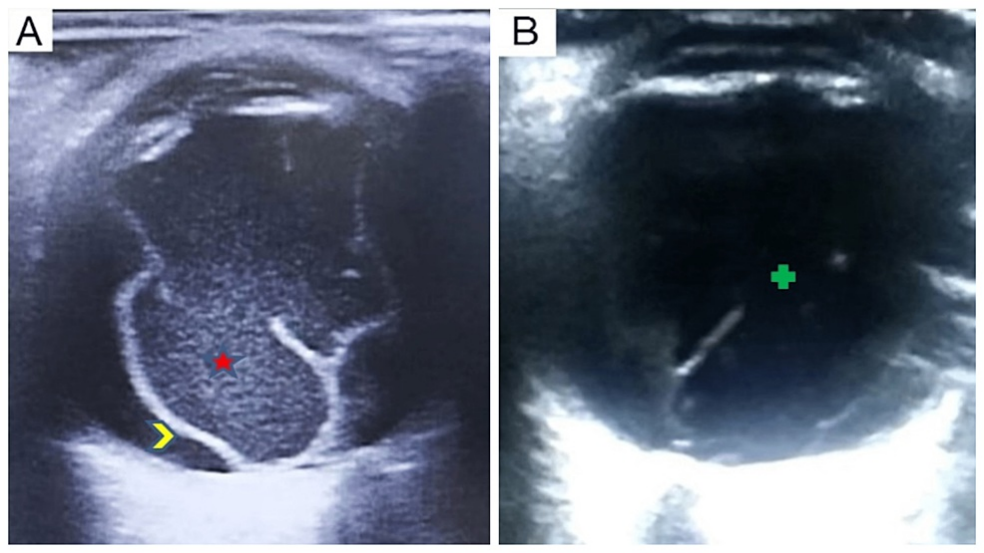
Ultrasound B-scan images of the posterior segment of the right eye. (A) On the third postoperative day showing multiple low to moderate reflectivity echoes in the vitreous cavity (red star) with an undulating moderately reflective membrane (yellow arrow-head) with good after movements suggestive of severe vitreous inflammation, posterior vitreous detachment and an attached retina. (B) At two weeks post-antiviral treatment showing significant resolution of vitreous echoes (green plus).
